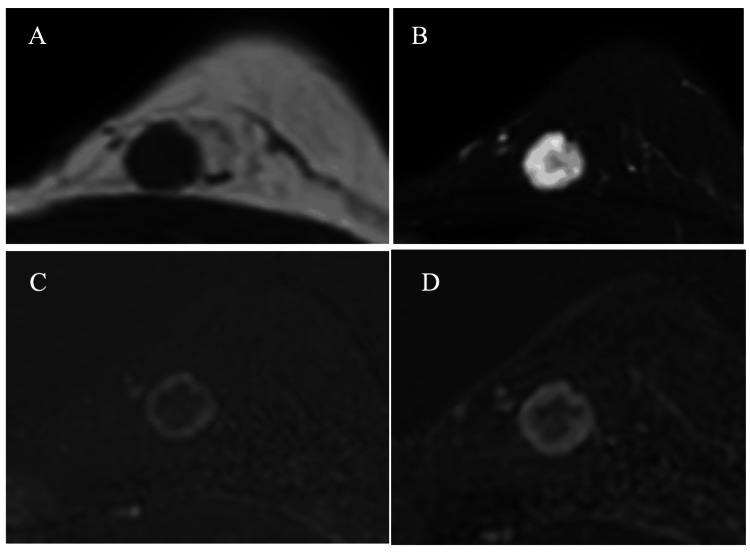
Magnetic resonance image (MRI) findings. MRI of the tumor showed an oval mass basically with low and high signals on (A) T1-weighted images and (B) T2-weighted images, respectively. The center of the tumor had somewhat low signals (B) and long-lasting rim enhancement, (C) early phase, (D) delayed phase on T2-weighted images and subtraction images, respectively.
